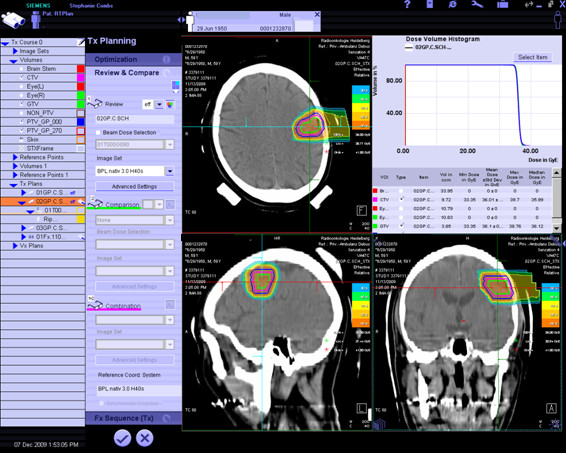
Typical treatment plan for carbon ion radiotherapy for a patients with a recurrent glioblatoma. Treatment is performed using the Siemens TPS for particle therapy, target volume delienation is based on CT- and MR-imaging with contrast enhancement.
